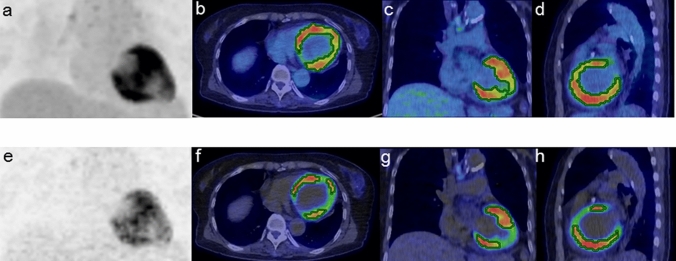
A 70-year-old female patient with CS who showed VT and cardiac dysfunction (EF: 40.0%) before treatment. The SUV images [maximum intensity projection (MIP) (a), transaxial (b), coronal (c), and sagittal (d)] and Ki images [MIP (e), transaxial (f), coronal (g), and sagittal (h)] both show the positive myocardium for which the VOIs were automatically determined. The SUV (SUVmax: 8.51 g/ml, SUVmean:4.66 g/ml, CMV: 220.0 cm^3^, CMA: 1025.2 g) and Ki parameters (Ki max: 370.2 × 10^–3^ ml/g/min, Ki mean: 214.9 × 10^–3^ ml/g/min, Ki volume: 115.3 cm^3^) were all positive for the risk of arrhythmic events according to each threshold criterion.
